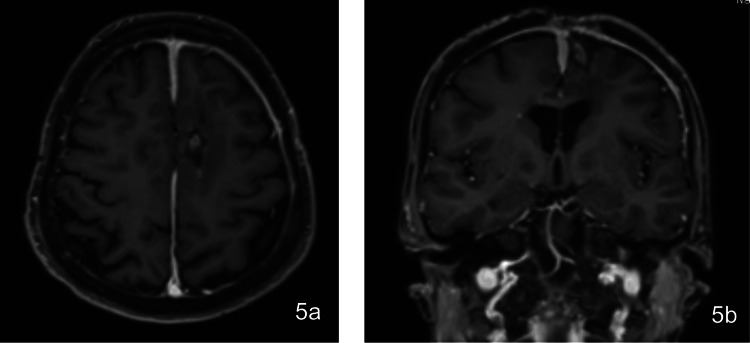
Postoperative MRI at two months in the (a) axial and (b) coronal planes showing closed calvarium with near resolution of cerebral abscess
